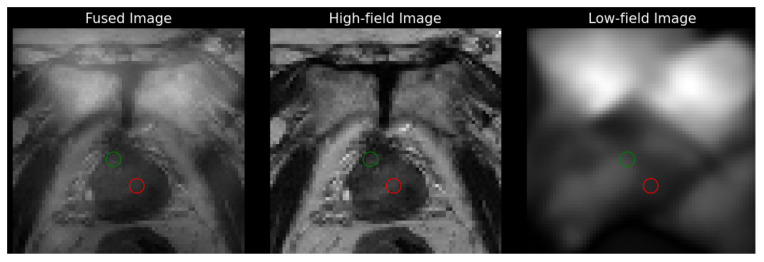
Co-registered, T2-weighted 3T and low-field MR images of a 77-year-old male with Gleason score 4+5 prostate cancer. The cancerous lesion (red) and a non-suspicious region with the same radius (green) are marked.
